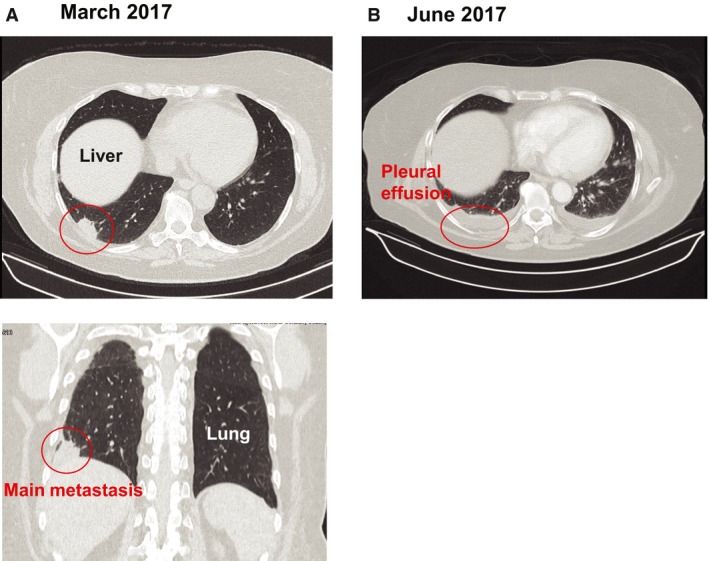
Preoperative CT scans of the urachal carcinoma patient. (A) CT scan in March 2017 showing a metastasis in the right lung. (B) CT scan in June 2017 depicting a pleural effusion before the surgery hiding a pleural carcinosis
